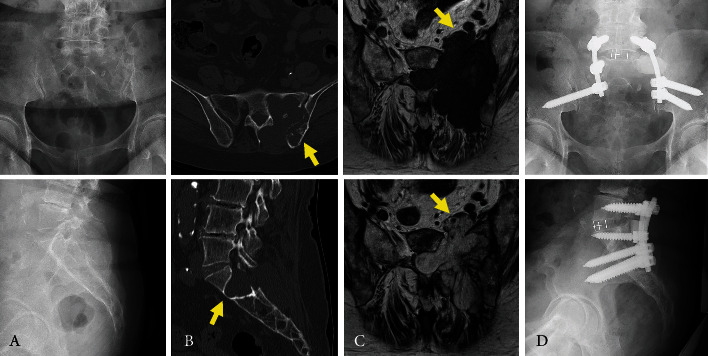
Chondrosarcoma in a 75-year-old woman. A plain radiograph showing a poorly specific finding in the sacrum (A). Computed tomography showing destruction of the bone in the left posterior ilium (arrow) ((B), top). The anterior cortex of the S1 vertebra was scalloped (arrow) ((B), bottom). Magnetic resonance imaging shows a lobulated lesion with homogenous low signal intensity on a T1-weighted image ((C), top) and high signal intensity on a T2-weighted image. The septal structure of each lobulated lesion had slightly low signal intensity on T2-weighted imaging ((C), bottom). The tumor is protuberant with a dumbbell shape in the enlarged S1 anterior foramen (arrows) (C). Plain postoperative X-ray radiograph, showing fixation of the L5-S-ilium (D).
